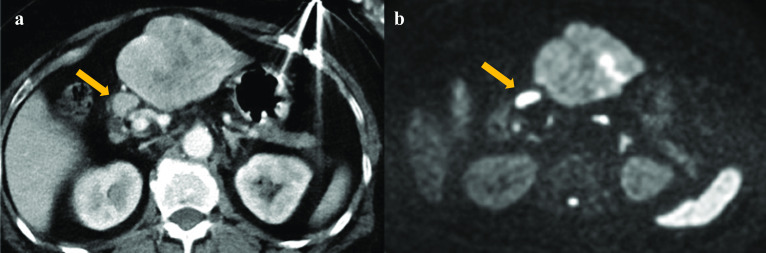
Lymphadenopathy on enhanced CT and diffusion-weighted MRI. A regional lymph node in the hepatoduodenal ligament was enlarged at 13 mm in size (a). The lymph node showed high signal on diffusion-weighted magnetic as well as liver tumors (b). It was suspected to be metastasis. *CT* computed tomography; *MRI* magnetic resonance imaging
